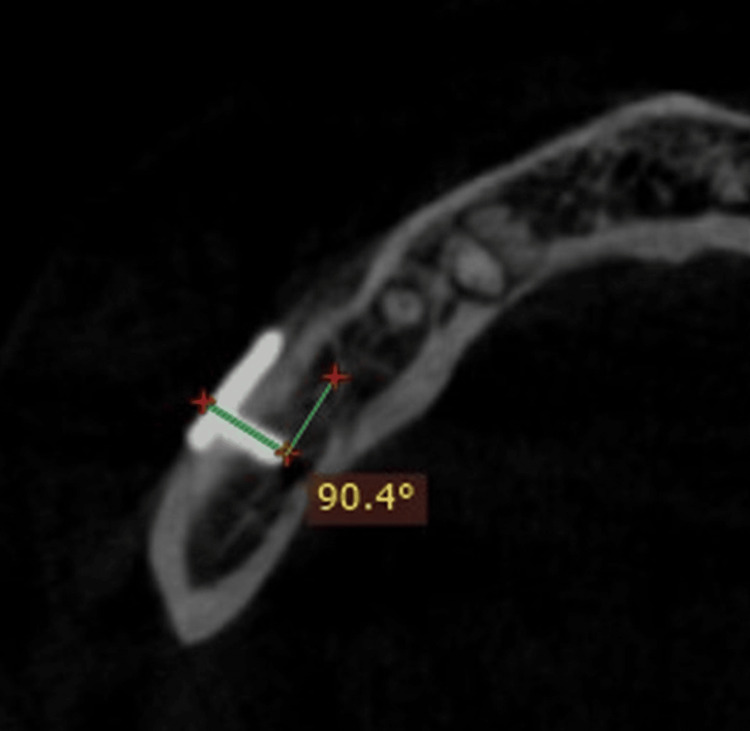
CBCT image showing angulation of screw fixation with a right-angled screwdriver CBCT, cone beam computed tomography
